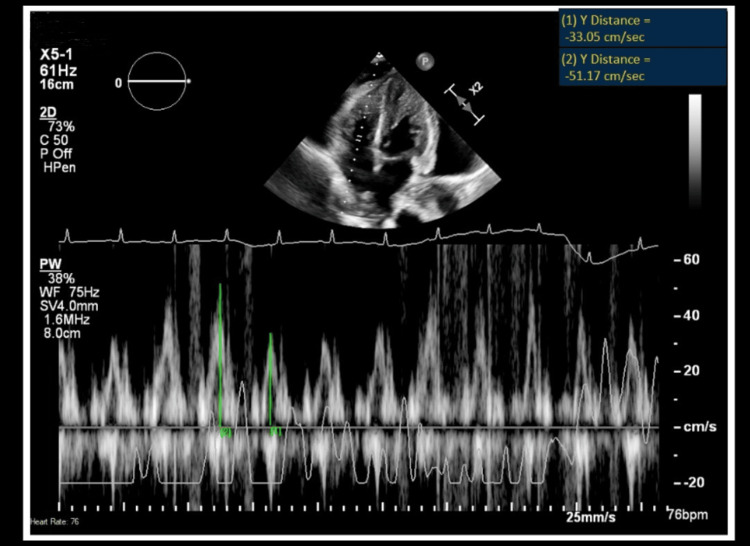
Pulsed-wave Doppler of tricuspid inflow demonstrating significant respiratory variation.
